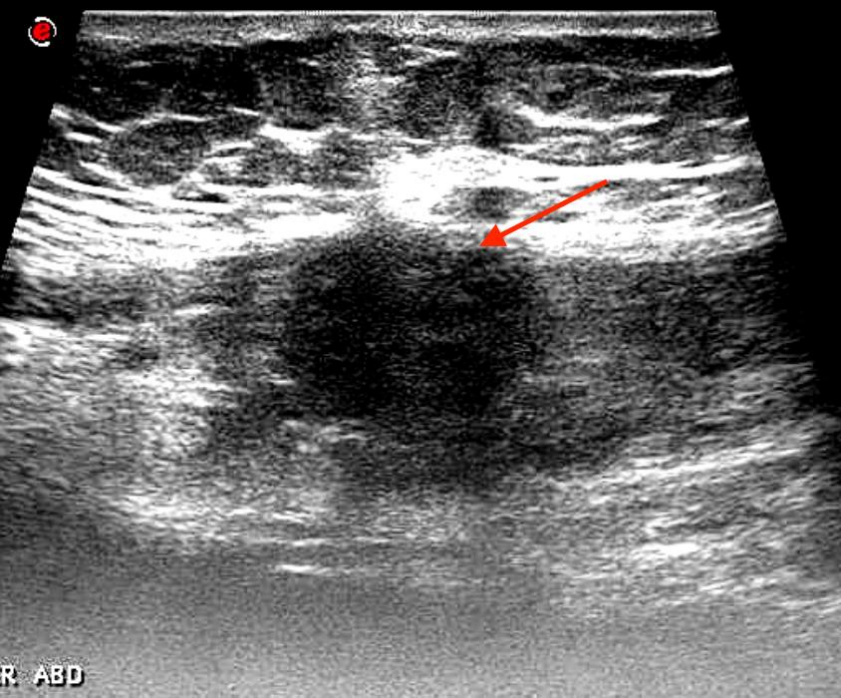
Ultrasound image of the endometrioma on left lower part of rectus muscle
